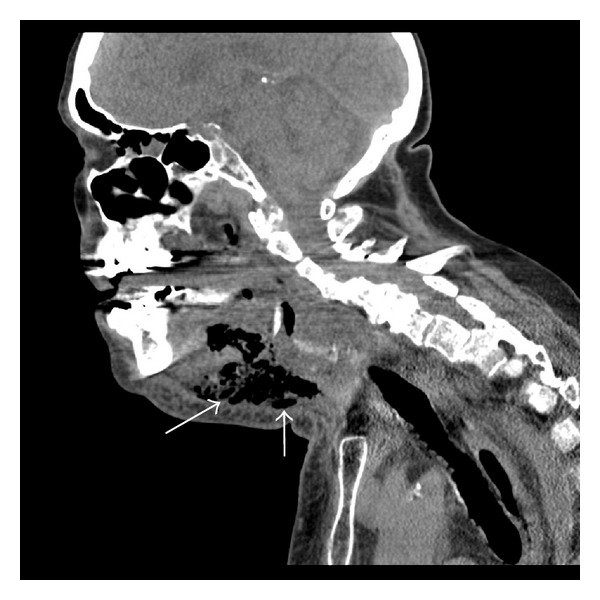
Sagittal CT image of patient preoperatively. Note the classic “sniffing” position of patient to maximize air entry in the presence of an upper airway obstruction. White arrows point to significant gas formation in anterior cervical space.
